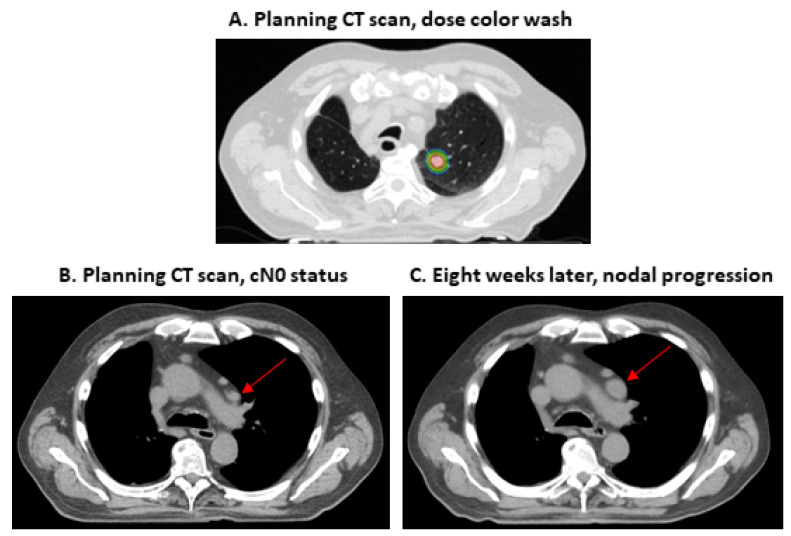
Clinical example of a patient without positron emission tomography/computed tomography staging and subsequent rapid regional progression. Male patient diagnosed with stage IA2 non-small cell lung cancer of the left upper lobe. The patient was staged with conventional imaging (computed tomography scan of the upper and lower body, cranial magnetic resonance imaging and bronchoscopy, image (B) shows the initial status). The patient was treated with 55 Gy in 5 fractions (prescribed to the 80% isodose; image (A) shows the dose from 55 Gy [blue] to 68.75 Gy [red]). A CT-scan 2 months later (image (C)) showed good response of the primary tumor, but revealed the progression of a mediastinal lymph node (growth from 1.5 cm × 0.9 cm to 2.3 × 1.5 cm, red arrows in images (B,C)).
